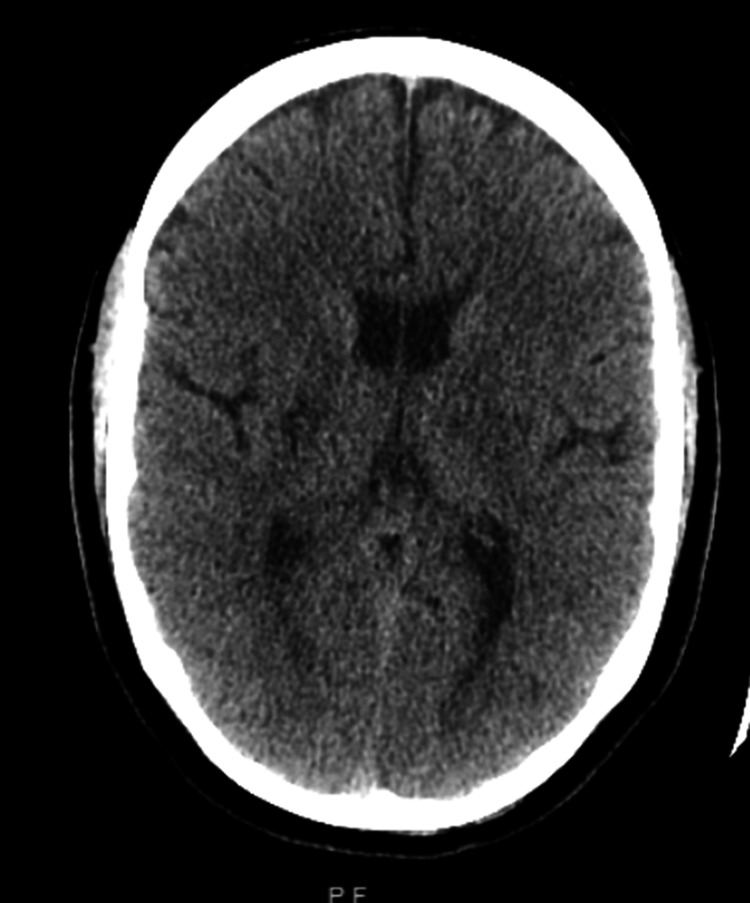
CT head upon presentation The image shows no evidence of intracranial hemorrhage or completed infarct compatible with current symptoms. There was evidence of a previous infarction of the right basal ganglia as reported in prior history CT: computed tomography
